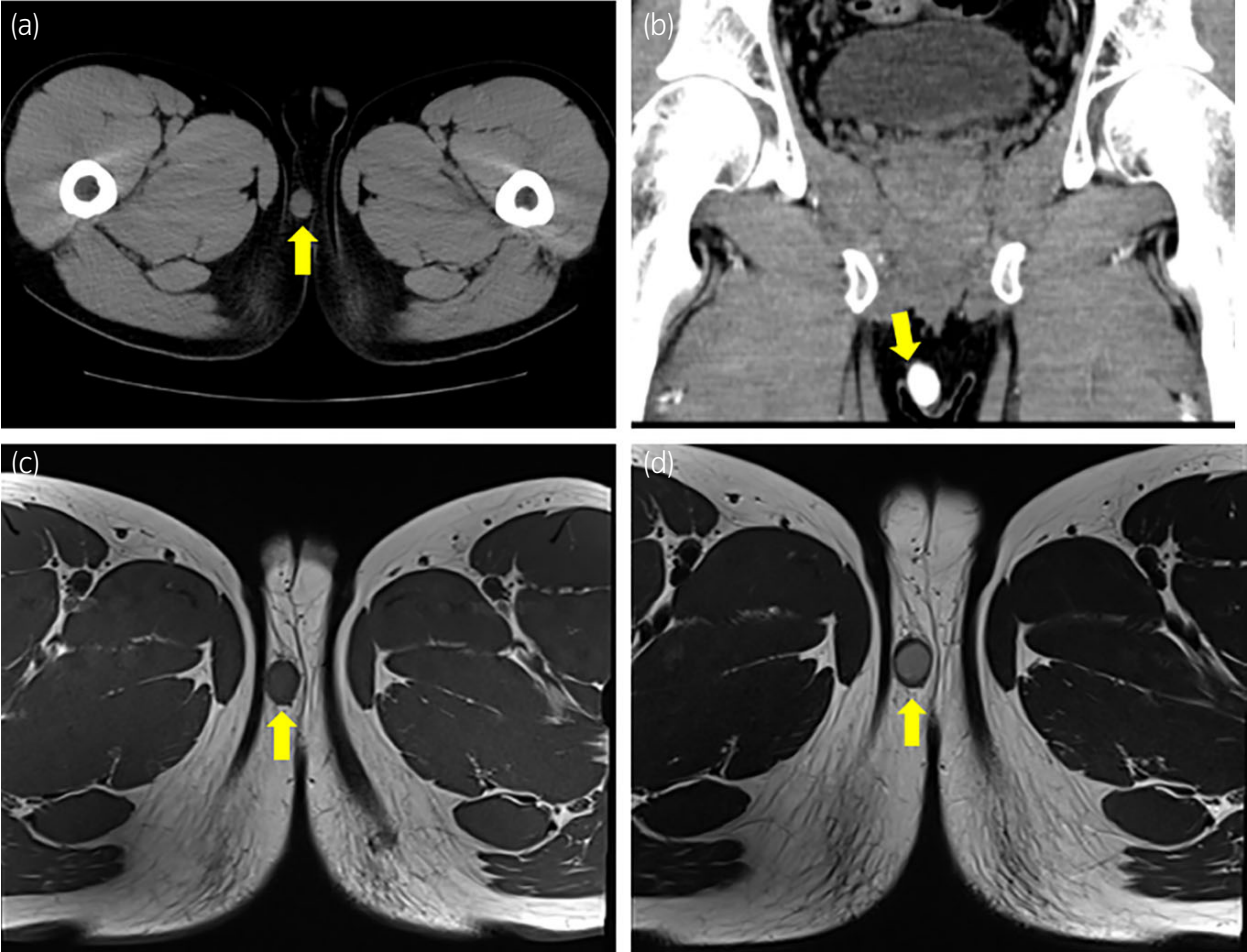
Radiological findings of perineal tumor. (a) CT shows a 20 mm subcutaneous tumor in the right side of the perineum. (b) Contrast ‐enhanced CT shows strong enhancement. (c) T1‐weighted MRI shows a well‐circumscribed mass with a mild hyperintensity. (d) T2‐weighted MRI shows a hyper‐intense mass
